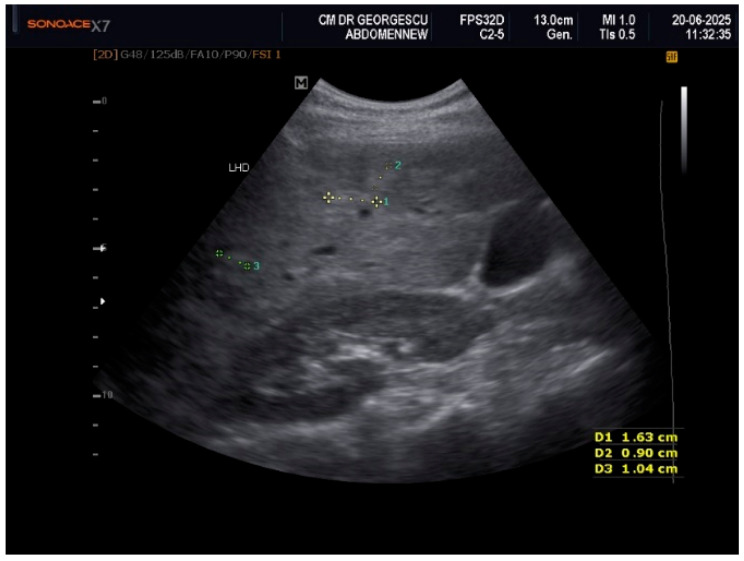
Two-dimensional ultrasound exam features of scattered hyperechoic nodules.
